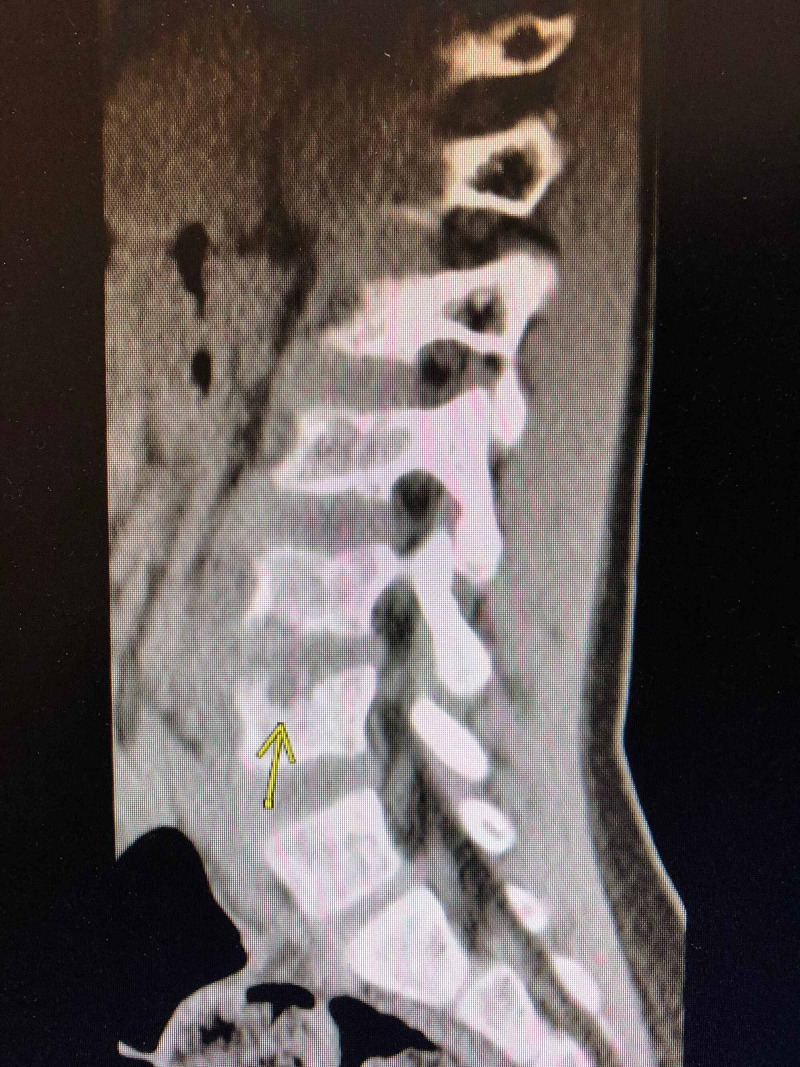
CT scan showing erosive changes in L5.
